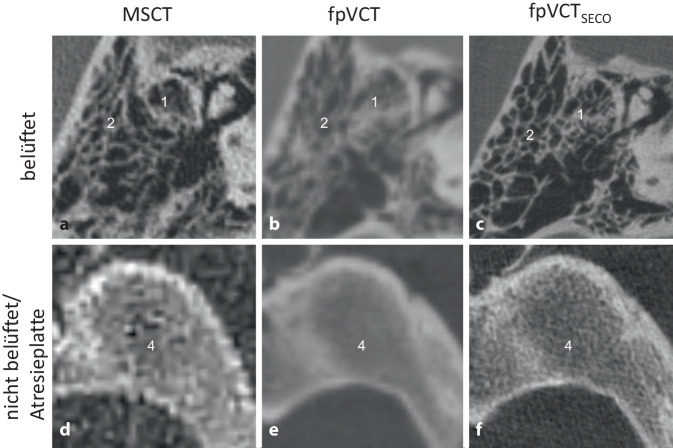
Visualisierung der Belüftung der Mastoidzellen. Ein repräsentatives Bild mit MSCT (600 µm Schichtdicke) wird in a gezeigt, eine Darstellung von fpVCT (466 µm Schichtdicke) in b und fpVCT_SECO_ (99 µm Schichtdicke) in c. d–f zeigen eine nicht belüftete/atresierte Platte. *1* belüftete Mastoidzellen, *2* knöcherne Atresie des äußeren Gehörgangs, *4* Mastoidatresieplatte, *MSCT* Multislice-Computertomographie, *fpVCT* „flat-panel volume computed tomography“, *fpVCT*_*SECO*_ sekundäre Rekonstruktionen der fpVCT
